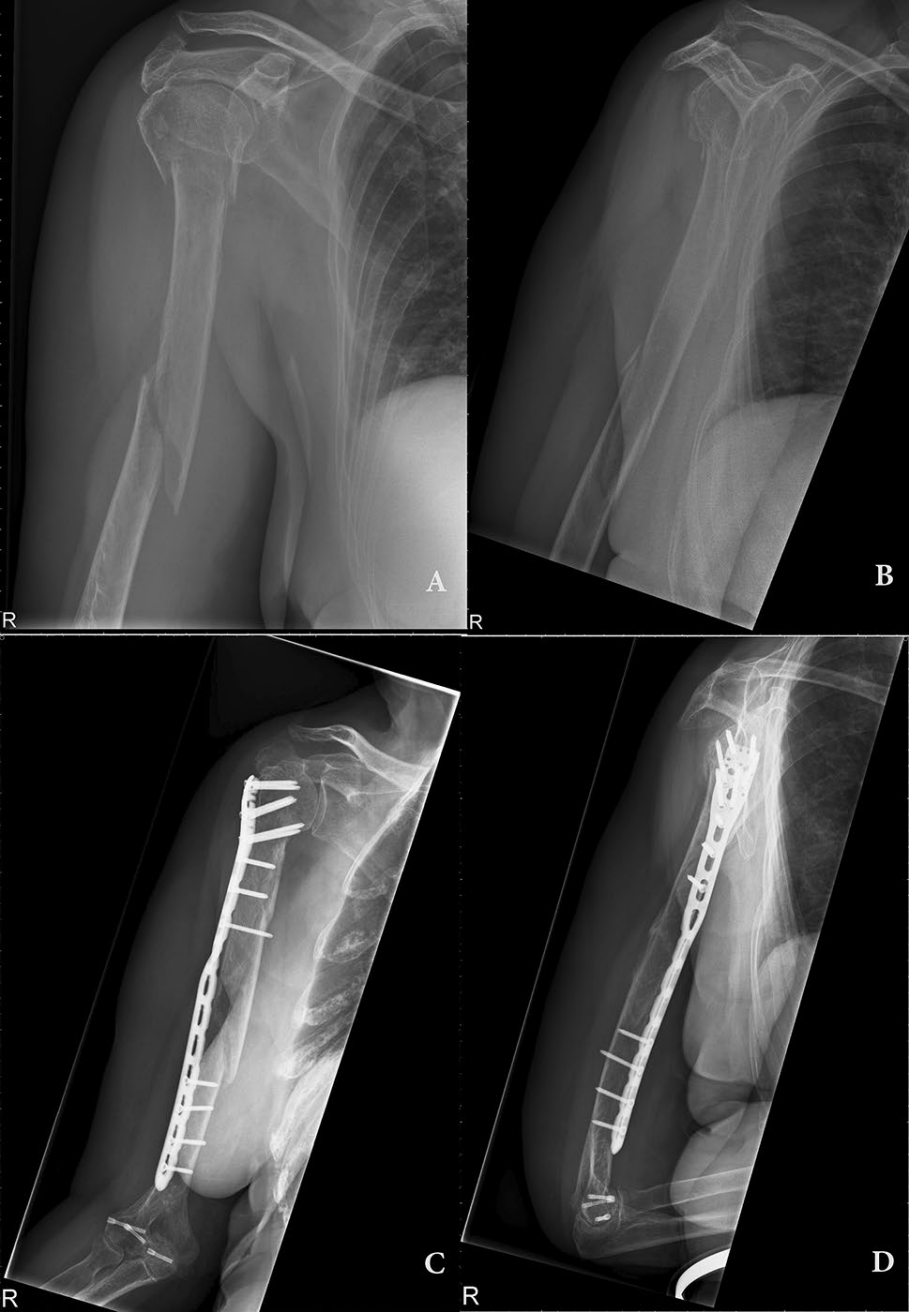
Clinical example of a pre-contoured helical plate in a female 79 years old patient. (A) and (B) preoperative X-ray of a proximal humeral fracture with meta-/diaphyseal extension. (C,D) Six month postoperative X-ray with a helical PHILOS plating system.
